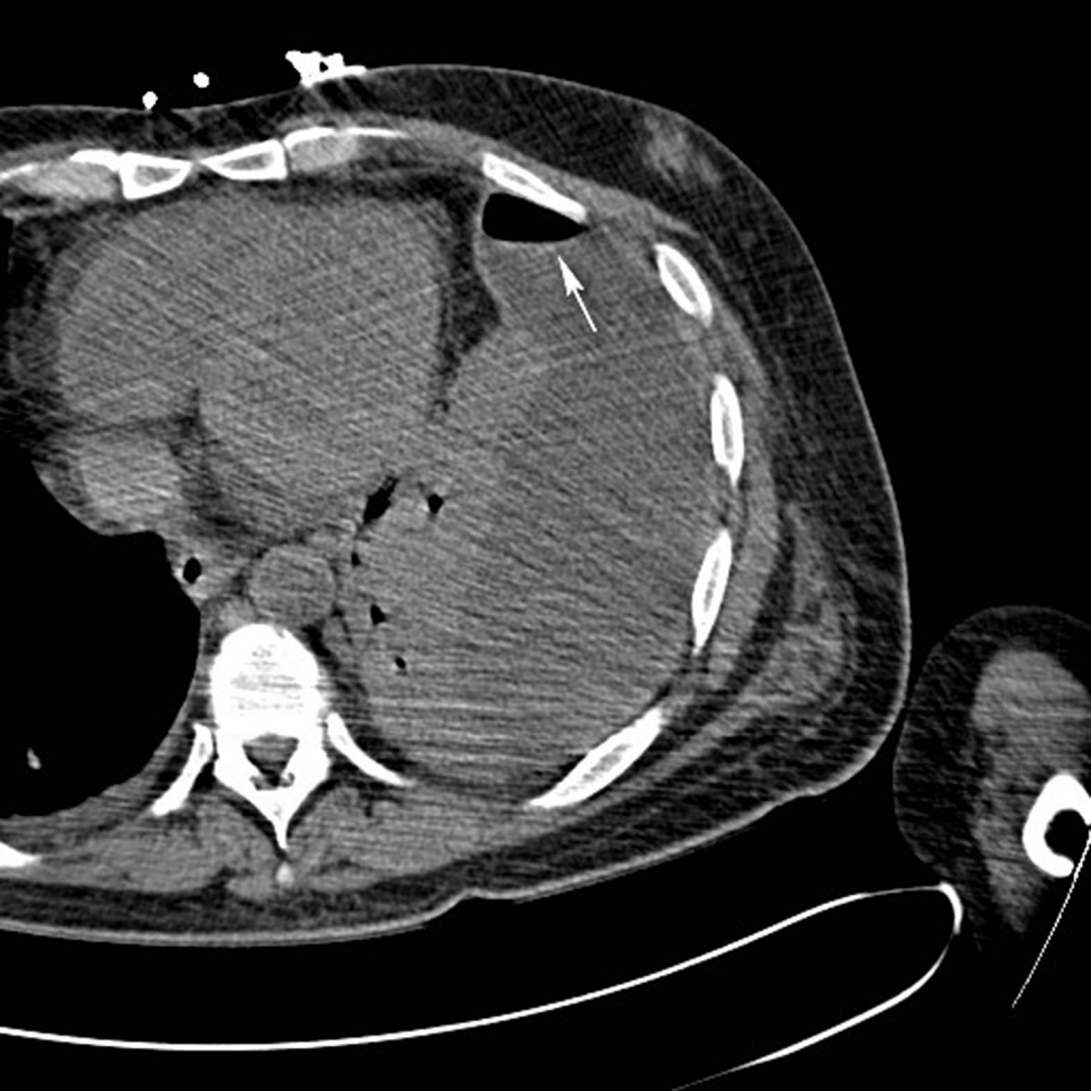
CT scan of the chest showing the loculated left pleural fluid collection with air-fluid level (arrow) and the adjacent parenchymal consolidation.
